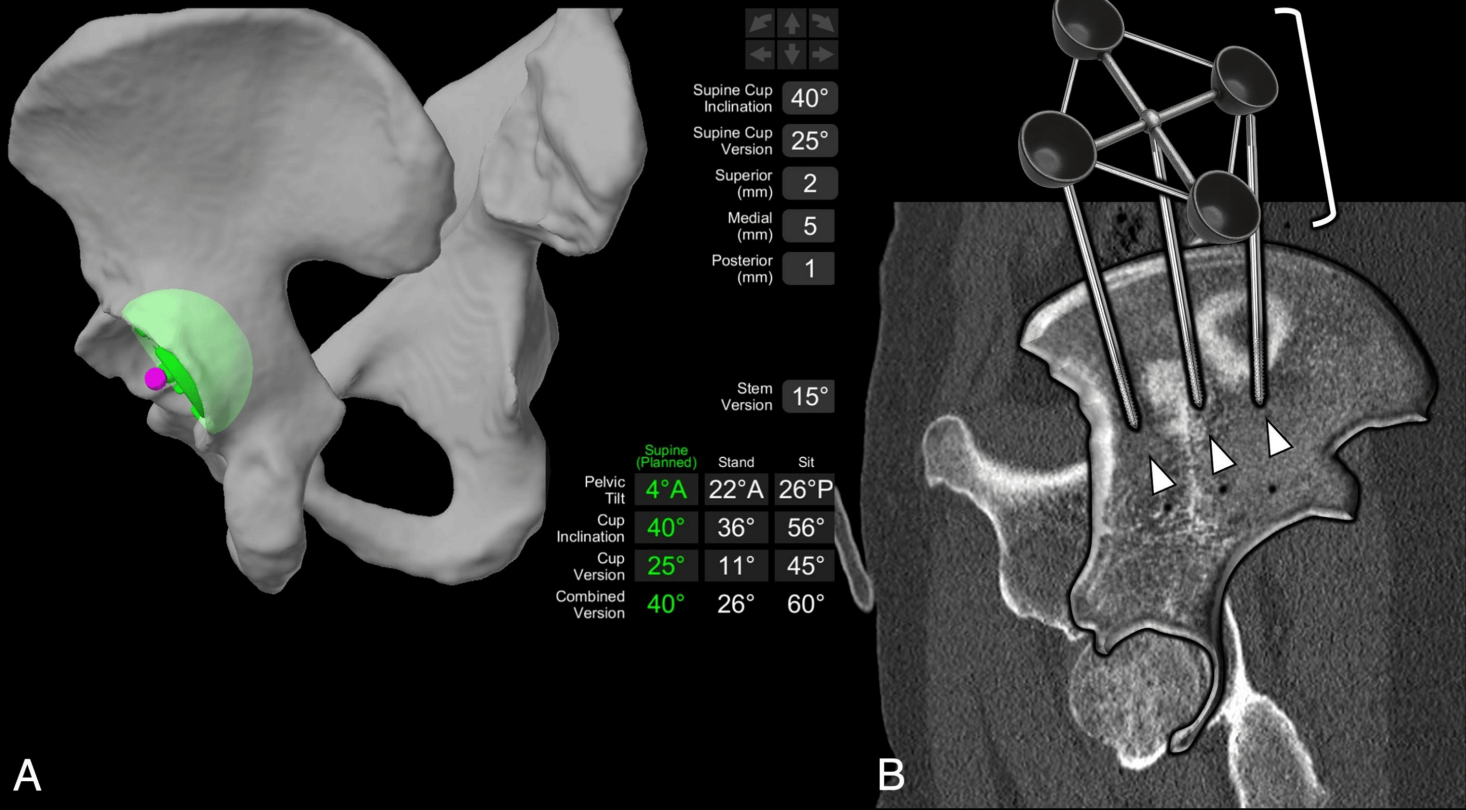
MAKO Robotic-Arm Assisted Surgery System (Stryker, Mahwah, NJ, USA) Preoperative CT-based planning. (A) Standing and sitting pelvic tilt, cup inclination, and version are loaded into the navigation system to ensure proper placement. (B) Pelvic array (reformatted and superimposed sagittal CT slices): three iliac pins (white arrowheads), placed posterior to the ASIS, anchor the pelvic array (white bracket) in place to provide spatial orientation for acetabular reaming, regardless of the patient's position. Image Credit: Juan Pablo Muñoz, corresponding author ASIS: anterior superior iliac spine
